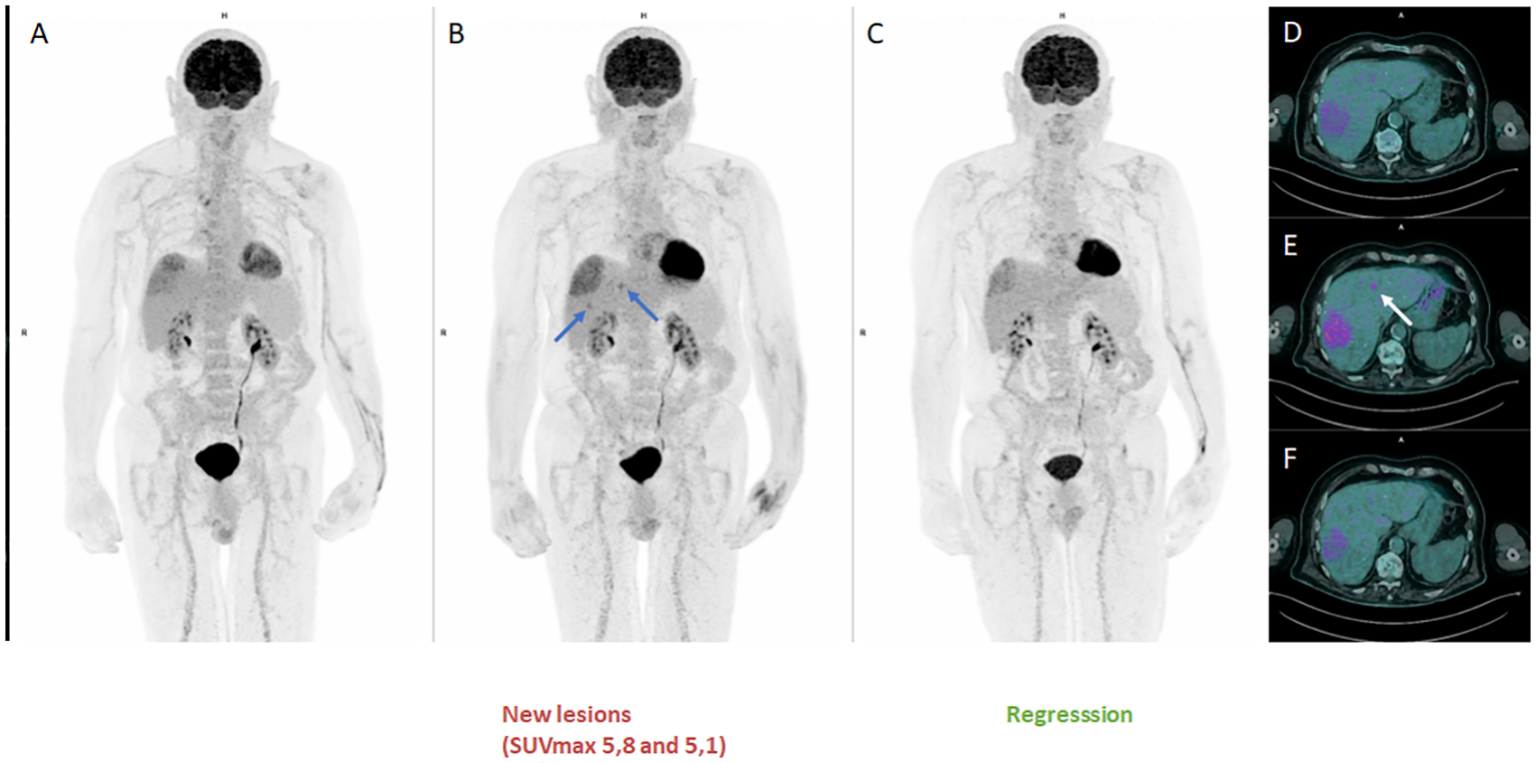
FDG-PET MIP third pseudoprogression (A, D: MIP and Axial disease stable before progression), (B, E : MIP and Axial disease progression according to PERCIST criteria with 27% increase in SULpeak of the main lesion and the recurrence of the 2 previous lesions), (C, F: MIP and Axial confirming a pseudoprogression (PsPD) with a stable SULpeak with renewed disappearance of the 2 hypermetabolisms seen on the previous scan.
